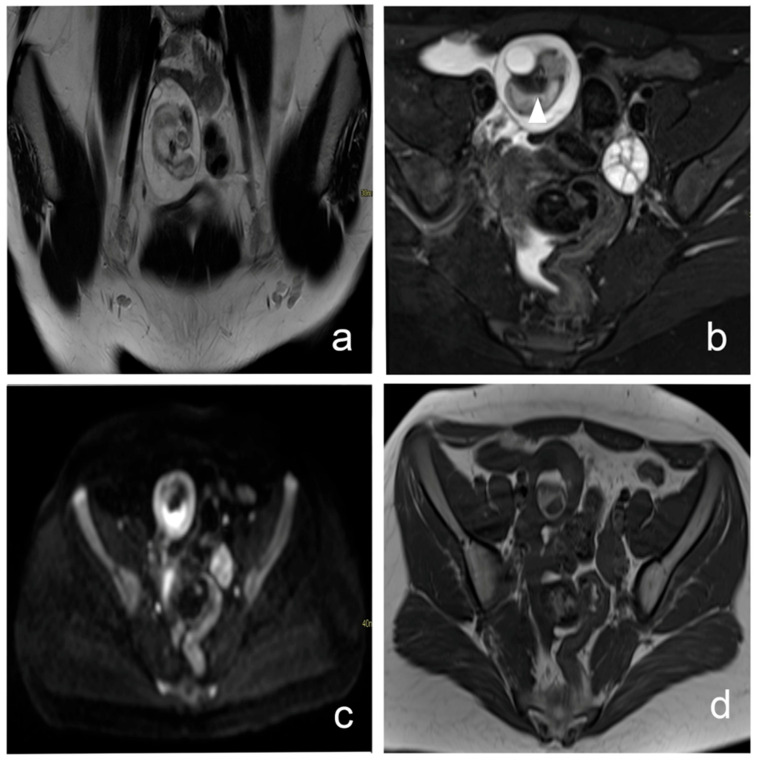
Ovarian torsion associated with a dermoid cyst in a 16-year-old female presenting with acute pelvic pain. (a) Coronal T2-weighted HASTE image shows an enlarged right ovary with stromal edema and multiple peripherally displaced follicles. (b) Fat-suppressed T2-weighted image shows suppression of the fatty component (head arrow) within the lesion. (c) Diffusion-weighted image demonstrates focal diffusion restriction corresponding to the Rokitansky nodule. (d) Axial T1-weighted image reveals hyperintense fat-containing components consistent with a dermoid cyst. A twisted vascular pedicle (“whirlpool sign”) and moderate pelvic free fluid are also visible. The presence of ovarian enlargement, stromal edema, and twisted vascular pedicle was consistent with ovarian torsion, which was subsequently confirmed at surgery.
